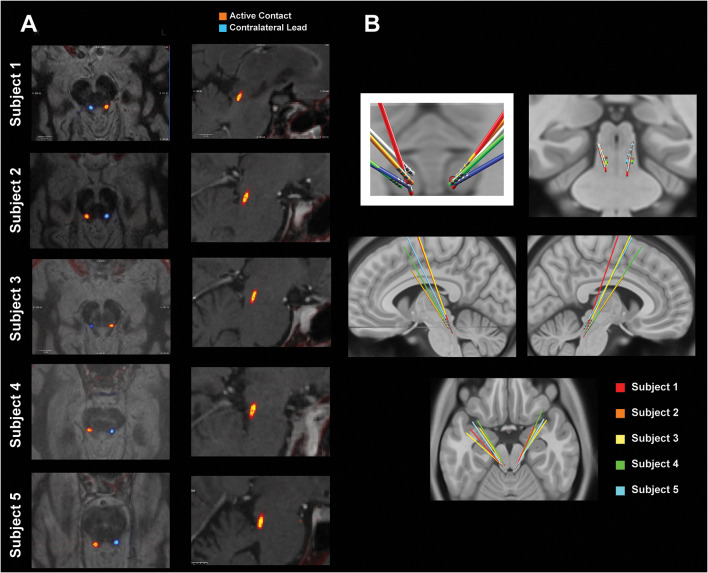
Pedunculopontine nucleus (PPN) lead localization methods. (A) MRI-CT co-registered images of each subject. The “active contact” marks the center of the bipolar electrode configuration that yielded the feature most correlated with gait. The left panels show T1 inverted axial views of the active contact (orange) and the contralateral lead (blue). The right panels show T2 sagittal views of the active contact. (B) Leads normalized to MNI space using Lead-deep brain stimulation (DBS). All leads are shown with a red dot that roughly defines the location of the PPN.
